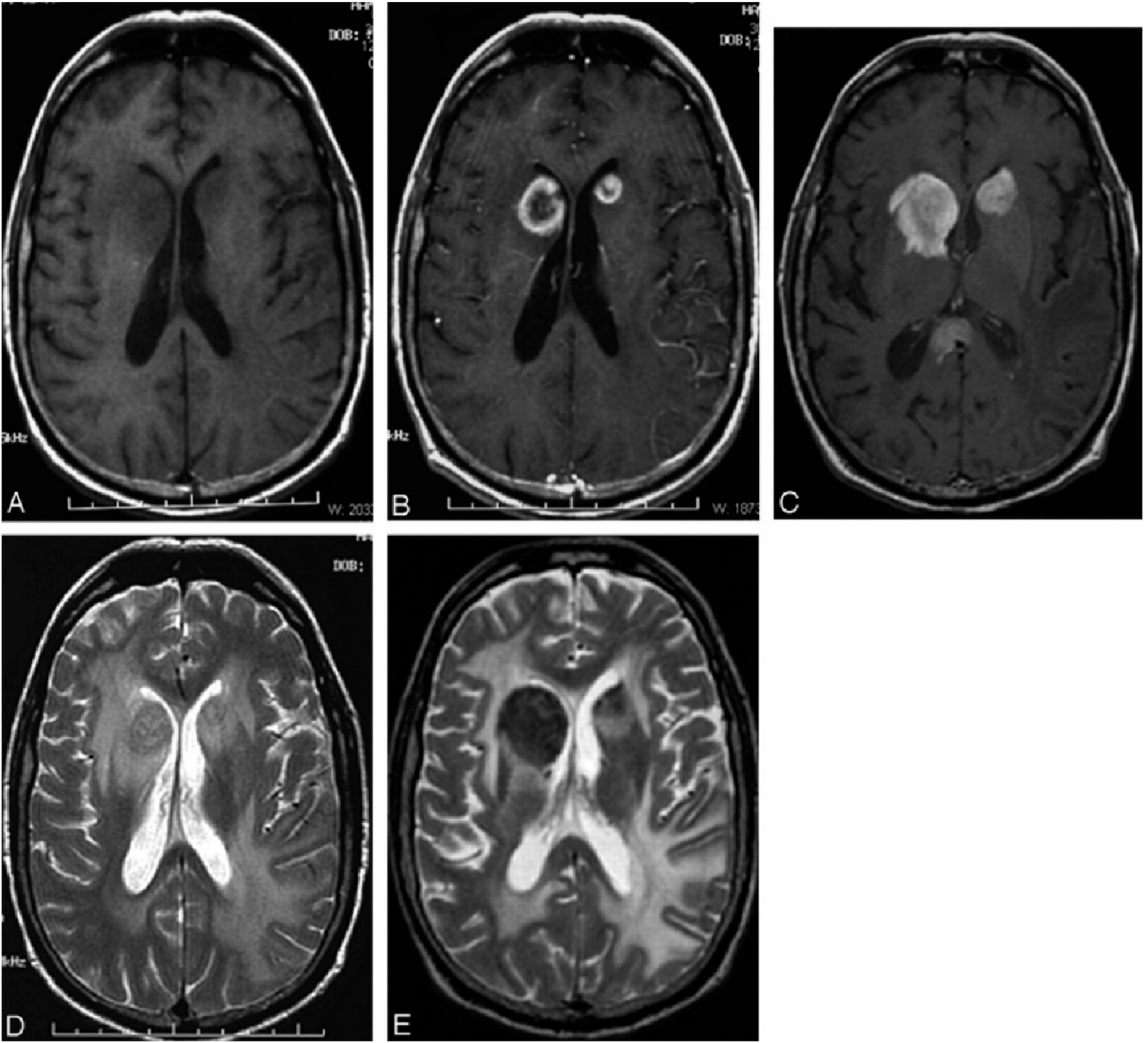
Patient with PCNSL. Axial T1-weighted images without gadolinium (A) and with gadolinium (B) reveal intense ring-enhancing lesions in the head of both caudate nuclei. Fifteen days later, T1-weighted images with ferumoxtran-10 (C) demonstrate that the lesions in the caudate head have enlarged and exhibit more intense enhancement. Additionally, another lesion in the splenium of the corpus callosum is visible, showing larger and more intense enhancement compared to the baseline gadolinium image (not shown). T2-weighted images following ferumoxtran-10 administration (D, E) display low-intensity changes in both lesions. Reproduction with the permission of reference (Manninger et al., 2005). Copyright ^©^2005 American Society of Neuroradiology Reproduction with the permission of reference (Manninger et al., 2005). Copyright ^©^ 2005 American Society of Neuroradiology.
